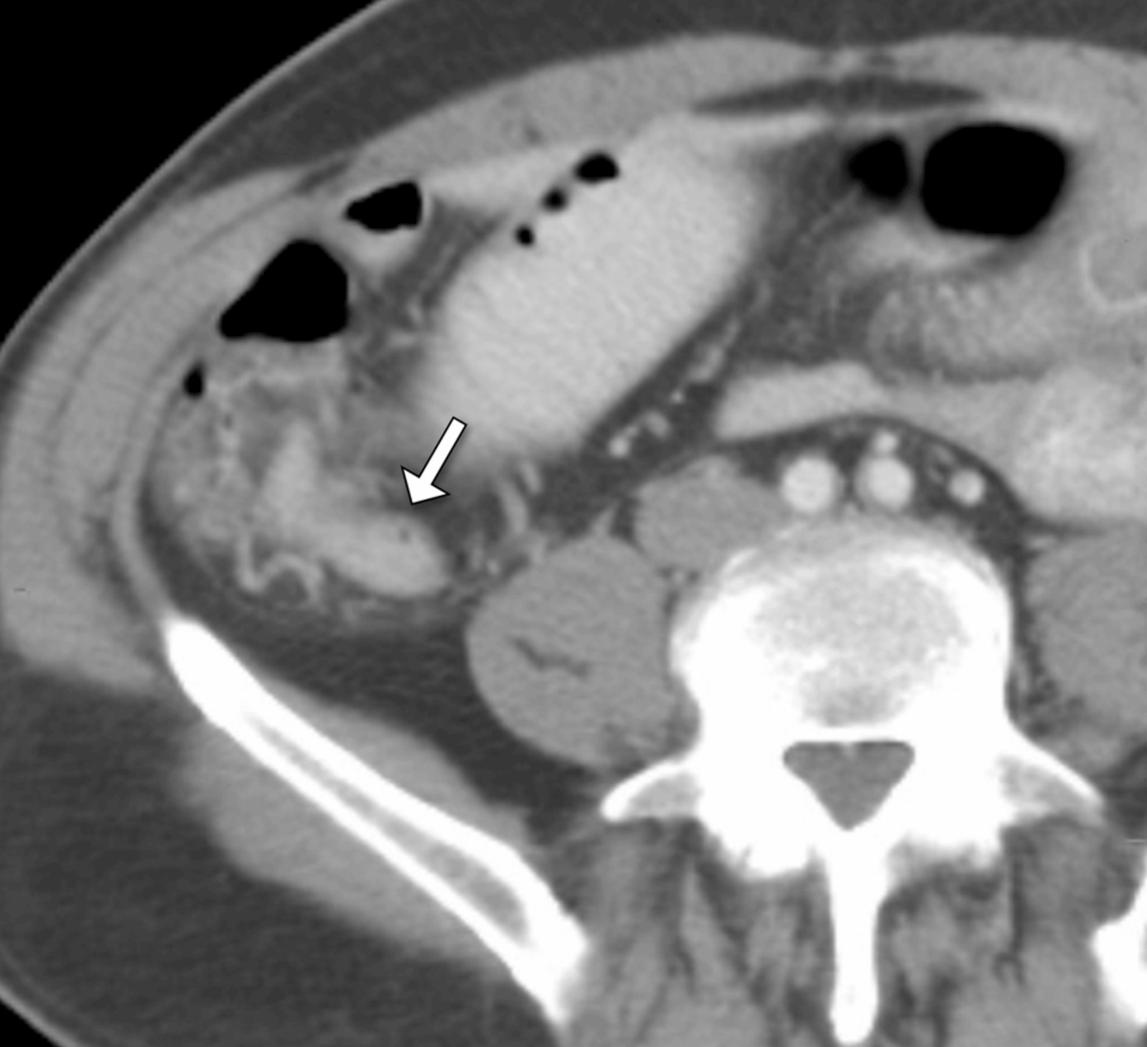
Axial CT showing terminal ileum thickening and stricture formation Axial contrast-enhanced CT of the abdomen demonstrates circumferential wall thickening and luminal narrowing of the terminal ileum (arrow), consistent with chronic ischemic stricture. No upstream dilatation of the small bowel is noted. CT: computed tomography.
